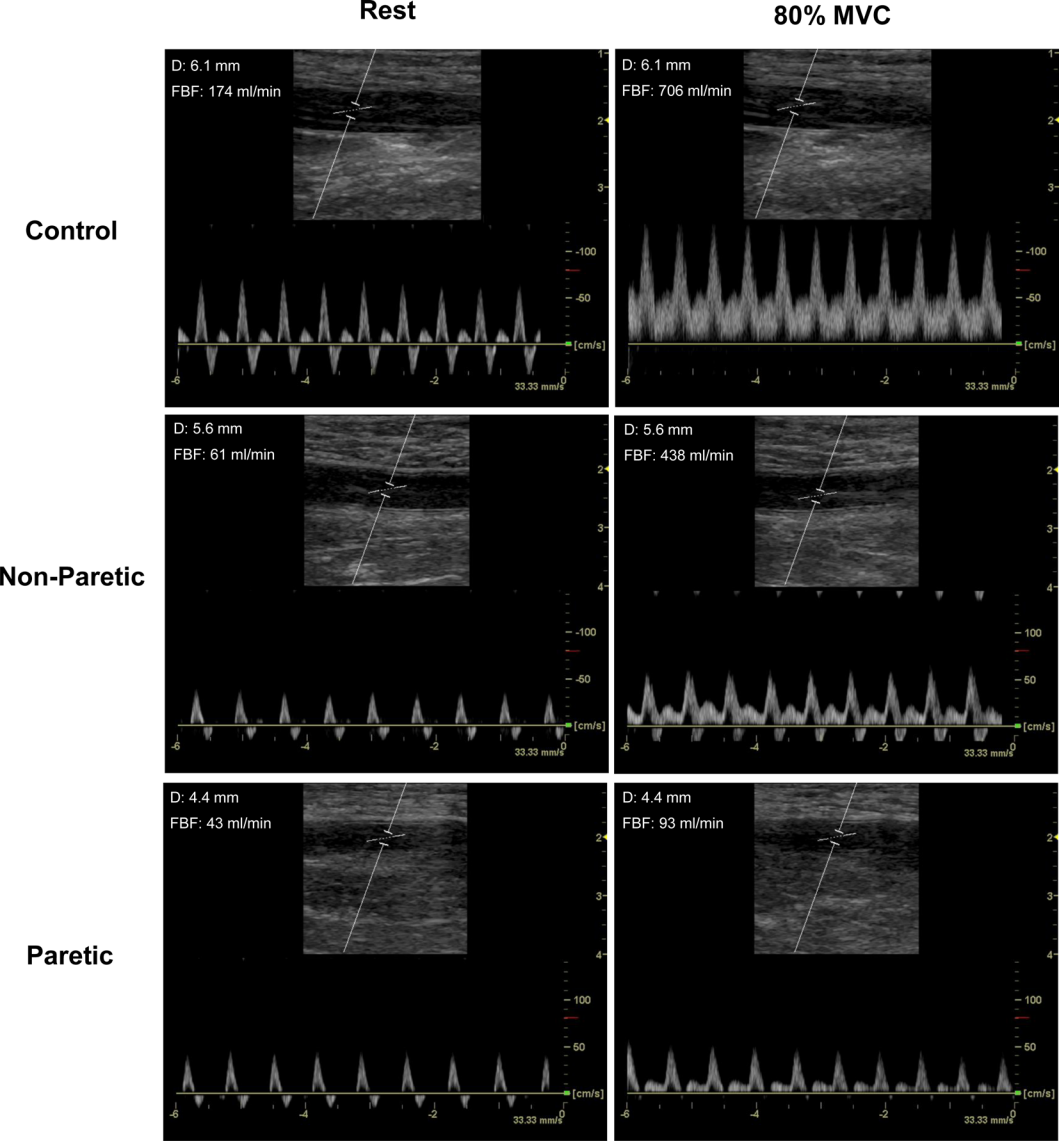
Representative ultrasound images showing blood flow through either the superficial femoral artery of a neurologically intact control subject or the paretic and non-paretic lower limb of a stroke subject at rest or immediately following an 80% MVC. Paretic and non-paretic superficial femoral artery images are from the same subject. D, diameter; FBF, femoral blood flow.
